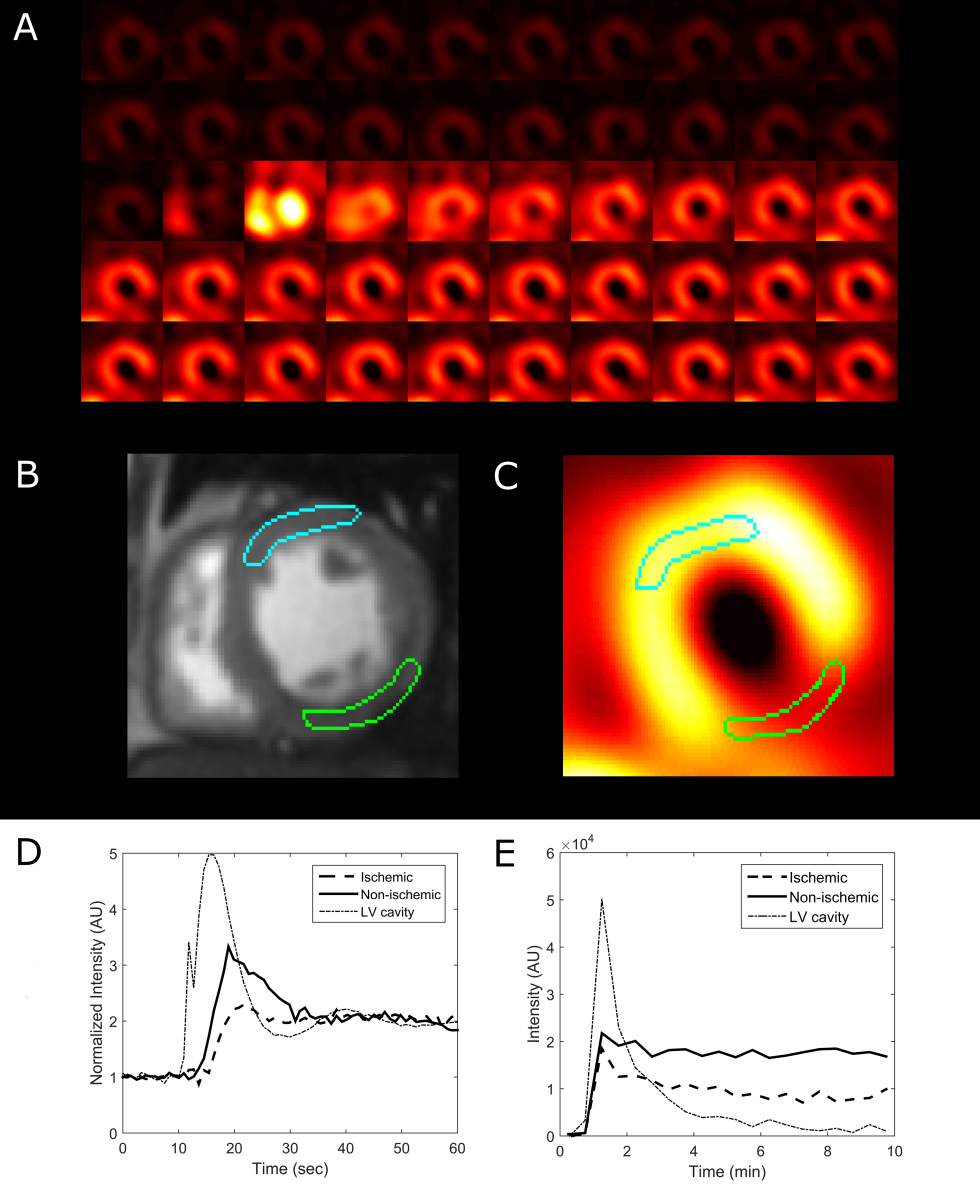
A representative case for flow quantification in a 70-year-old woman. (A) Dynamic SPECT images of a short-axis slice. During the second injection, the bright spots represent the left and right ventricular cavities. (B) The MR slice and ROI placement over the ischemic (green) and non-ischemic (blue) regions. (C) ROI placement of the SPECT image. (D) Time-intensity curves of the LV cavity, and ischemic and non-ischemic ROIs derived via MRI. (E) Time-intensity curves of the LV cavity, and ischemic and non-ischemic ROIs derived via SPECT. In the ischemic region, the hyperemic MBF was found to be 0.73 using MRI and 0.82 using SPECT. In the non-ischemic region, the hyperemic MBF was found to be 2.06 using MRI and 1.65 using SPECT. CAG indicated a 92% stenosis in the mid-LCX.
